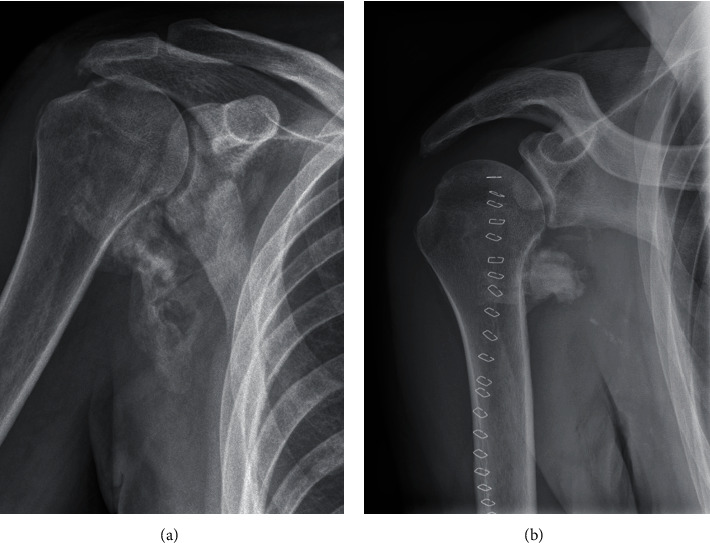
(a, b) AP radiograph demonstrating the extent of the HO one week before (a) and two days after (b) surgery. A small residual of the HO remained (b).
